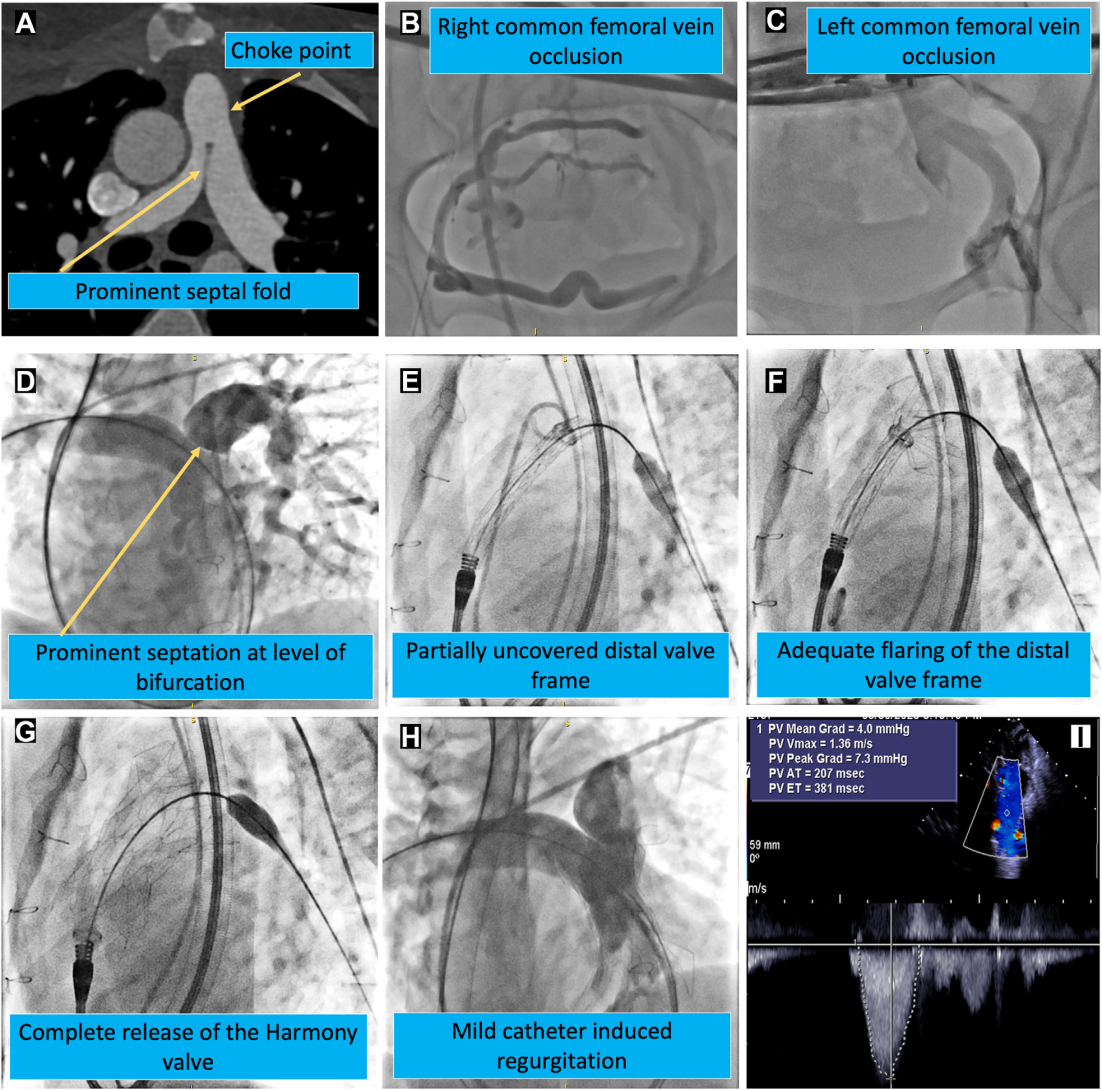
(A) Computed tomography demonstrates prominent septal fold proximal to the pulmonary artery bifurcation. (B,C) Bilateral common femoral vein occlusion. (D-G) Deployment of Harmony valve under angiographic guidance. (H) Repeat angiogram demonstrates mild catheter-induced regurgitation. (I) Intracardiac echocardiography demonstrates normal leaflet mobility with no regurgitation.
